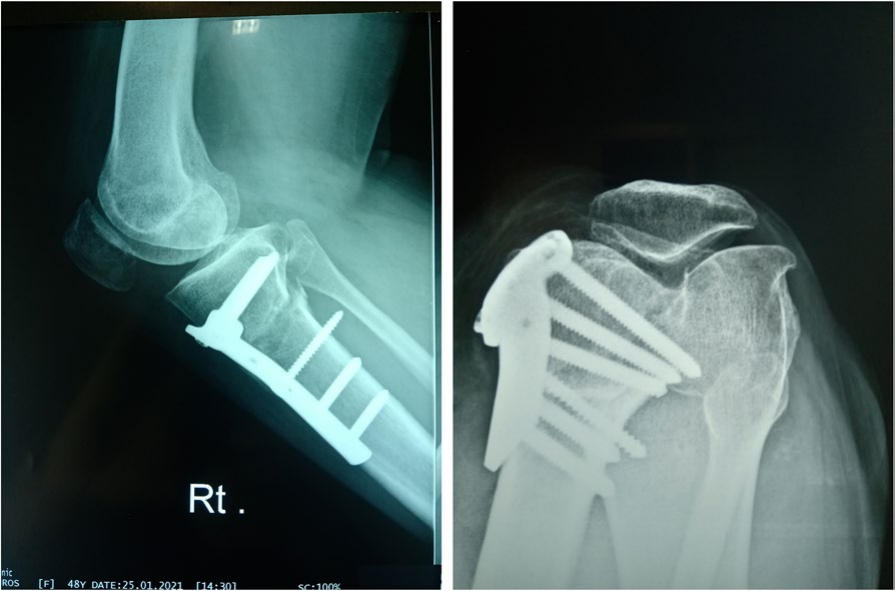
Postoperative x-ray: skyline and lateral views
